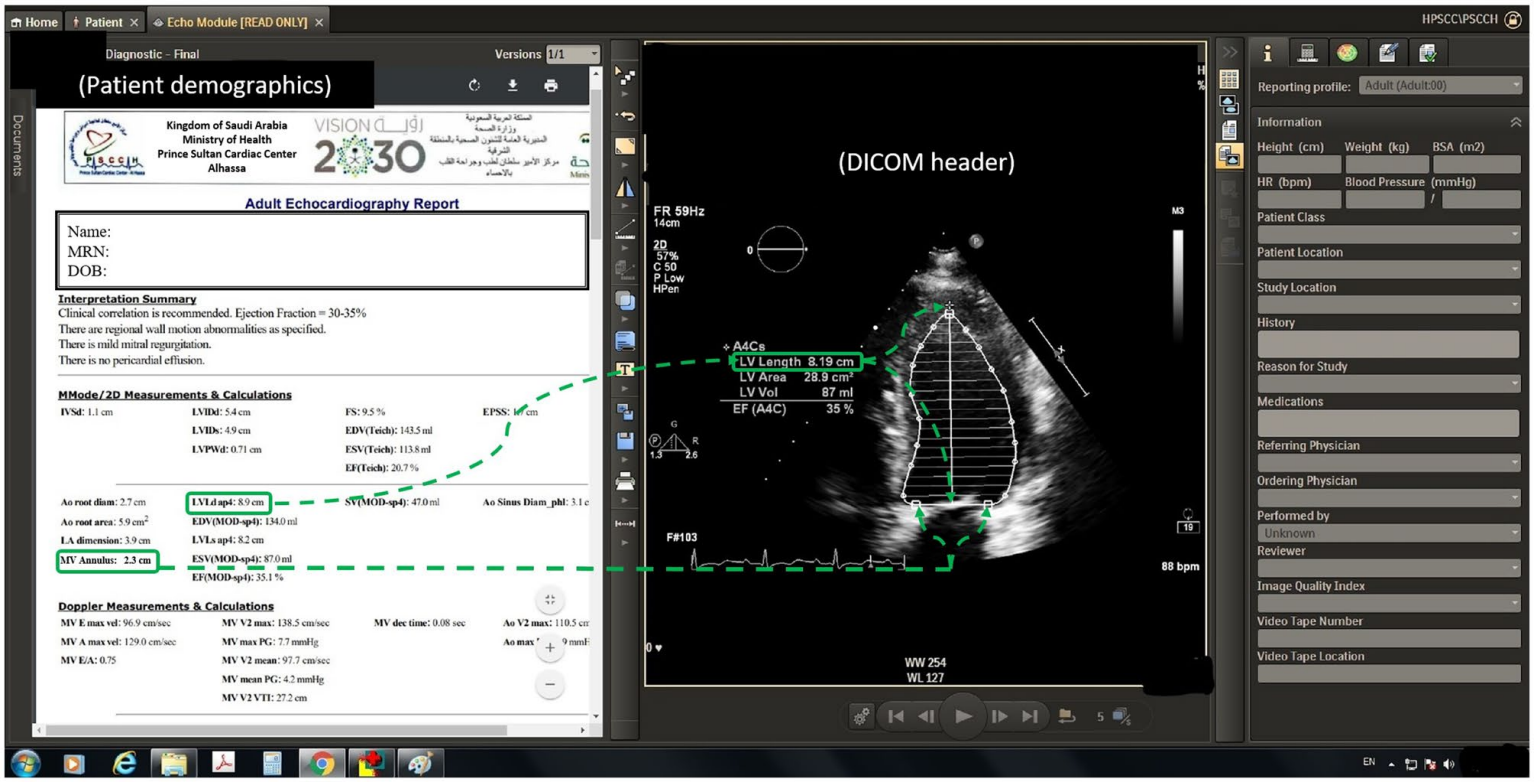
Mock-up echocardiogram interactive multimedia report incorporating multimedia elements and structured text. When the user clicks on report structured text measurements in a future interactive report, the hyperlink could open the relevant cine frames and annotation, in this case the left ventricular length or mitral valve annulus diameter (dotted arrows)
